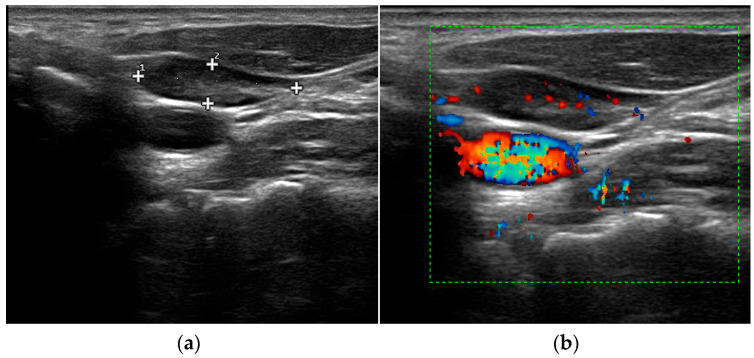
Grey-scale (a) and Color Doppler (b) aspect of cervical lymph node.
